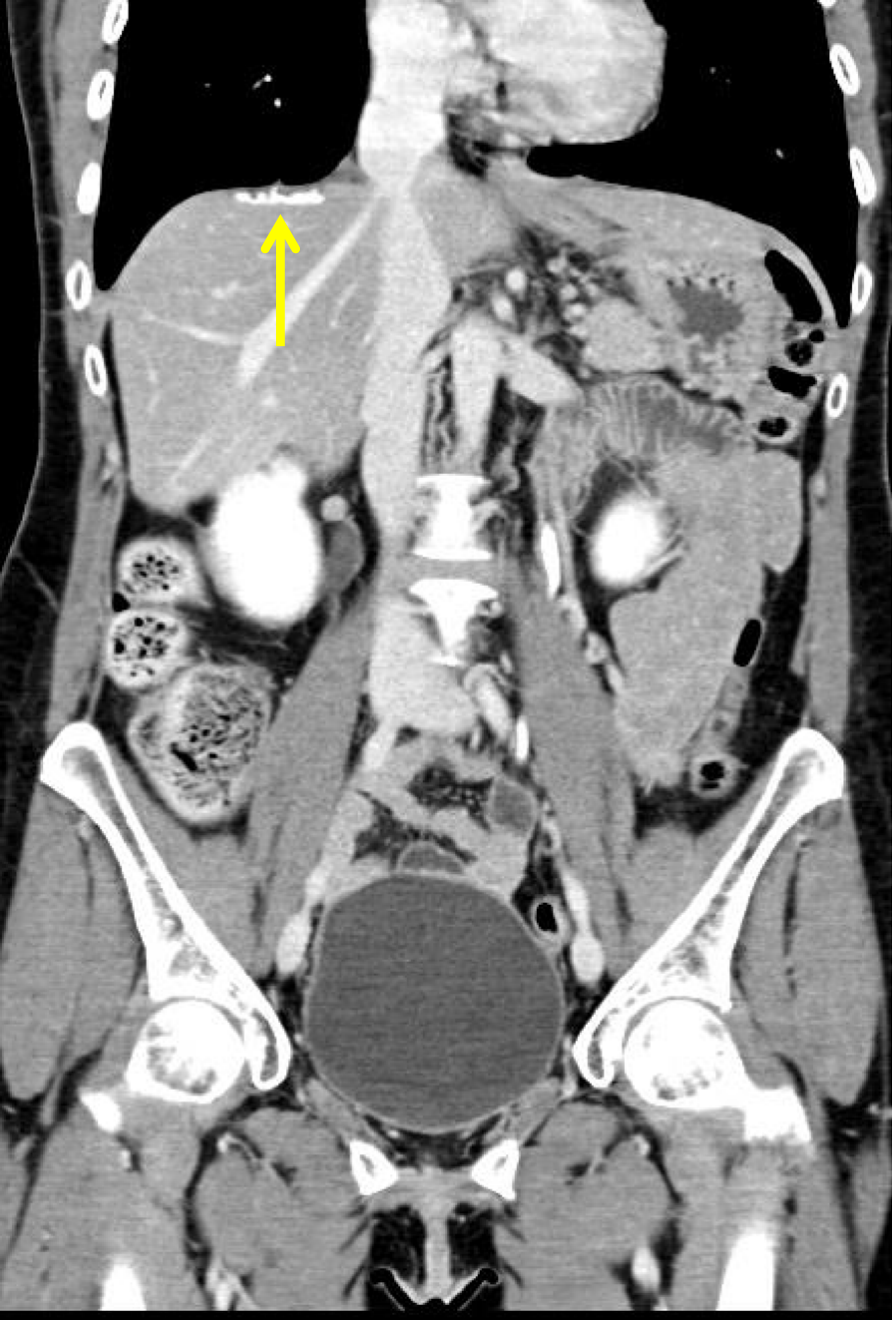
Computed tomography scan of hyperdense lesion in the right subdiaphragmatic region and a plaque thickening of the diaphragmatic dome (arrow).
